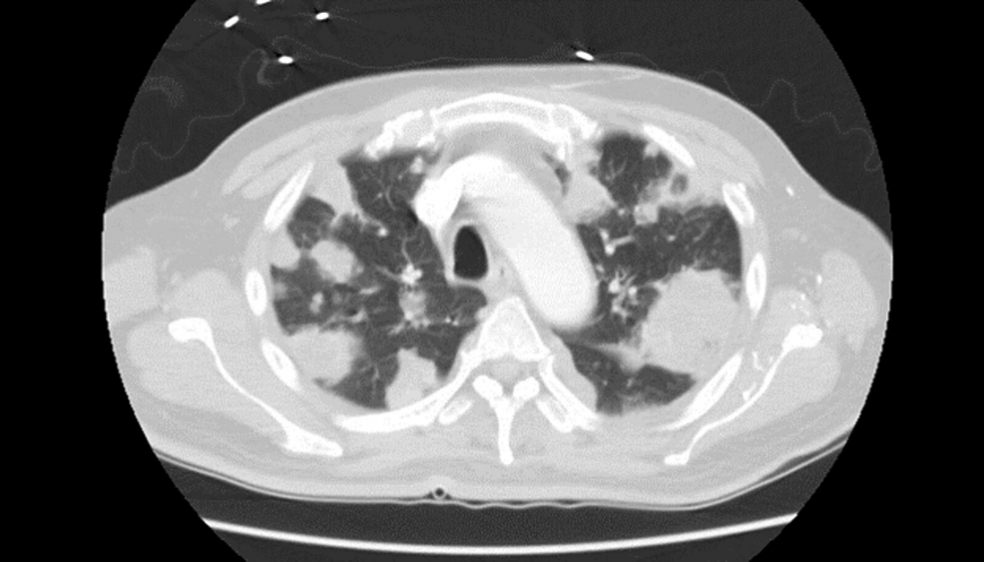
Coronal cut of CT chest showing multiple rounded masses and consolidations CT: computed tomography
